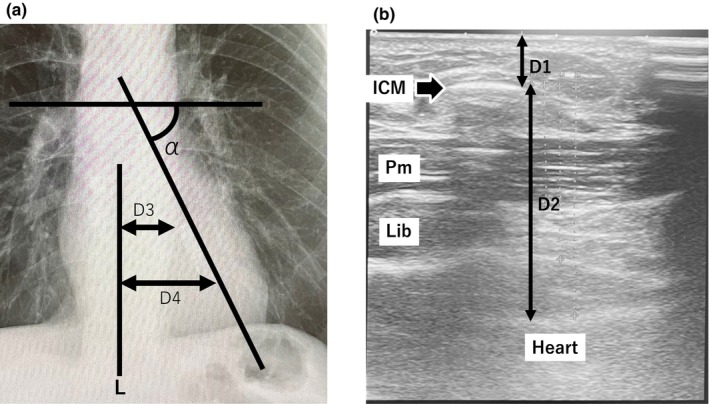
Images for measuring the position of the implantable cardiac monitor (ICM). (a) Chest radiograph. The proximal (D3) and lateral (D4) distances were the distances from the median line (L) and proximal and distal margins of the ICM. The angle was measured along the electrical axis of 12 leads (α). (b) Ultrasound image measuring the depth of the ICM. (D1); distance between the body surface and the ICM (D2); distance between the ICM and the heart surface. ICM, implantable cardiac monitor; Pm, pectoralis major muscle.
